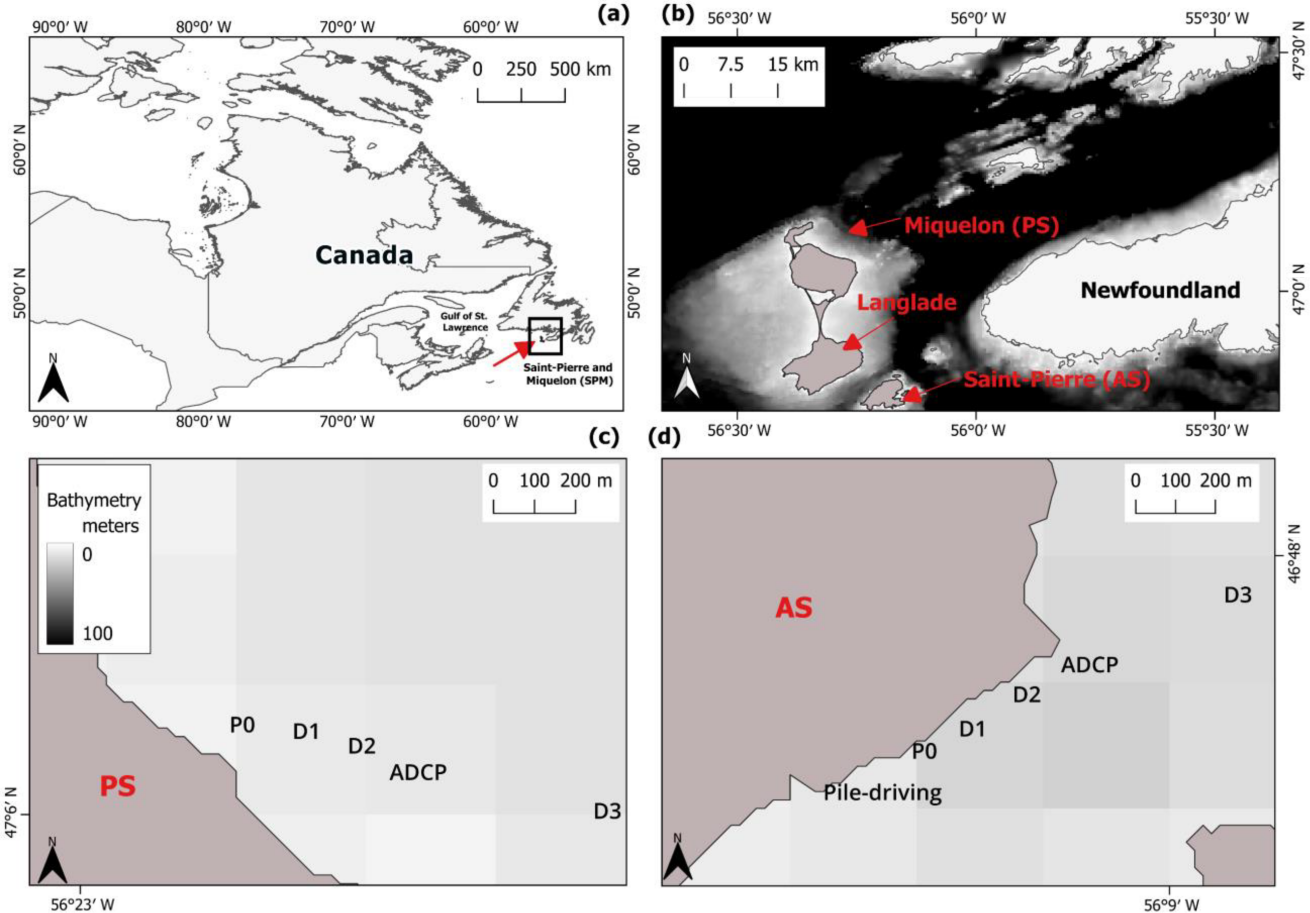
Set of maps of our study area: (a, b) Location of Saint‐Pierre and Miquelon's archipelago, where each site is referred as pristine (PS) or anthropized (AS) due to the level of anthropogenic noise at the islands of Miquelon and Saint‐Pierre, respectively. (c, d) Bathymetric maps containing sites, and stations from the sound (D1, D2, and D3) on transects exposed to an anthropogenic noise source (P0) at pristine site (PS) and anthropized site (AS), which had pile driving operations occurring at the same time of sampling. Bathymetry data were retrieved from General Bathymetric Chart of the Oceans (GEBCO). Bathymetric maps are composed using grayscale where white represents 0–1 m, 2–30 m light to dark gray, and values >100 m are black. ADCP, acoustic Doppler current profilers.
